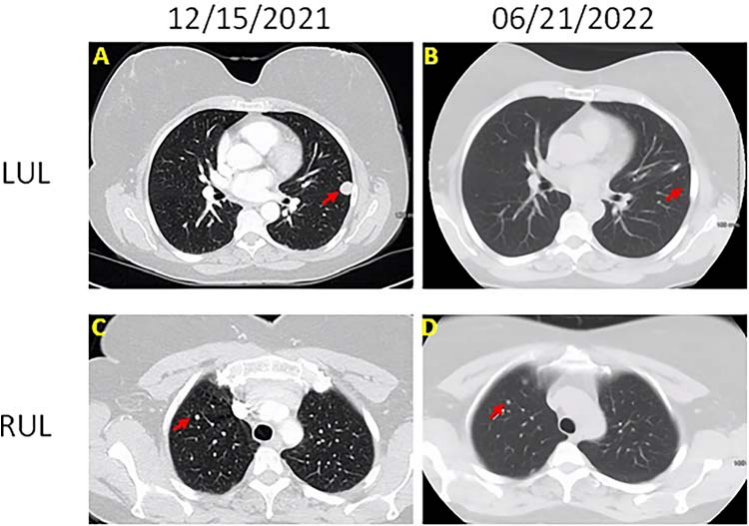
Chest CT images of the lesions (A) left upper lobe lesion (1.5 cm) before surgery on 15 December 2021; (B) left upper lobe lesion after surgery on 21 June 2022; (C) right upper lobe lesion (0.5 cm) on 15 December 2021; (B) right upper lobe lesion on 21 June 2022; there is no change on the size.
